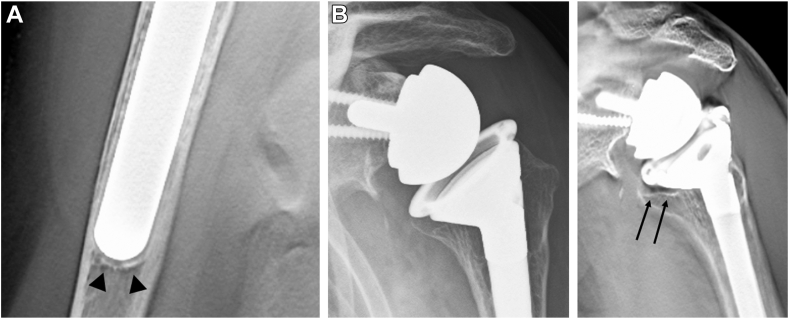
(A) Condensation line in zone 4. (B) The plain radiograph does not show the osteolysis, but the tomosynthesis clearly shows the osteolysis. *Arrow*: osteolysis.
